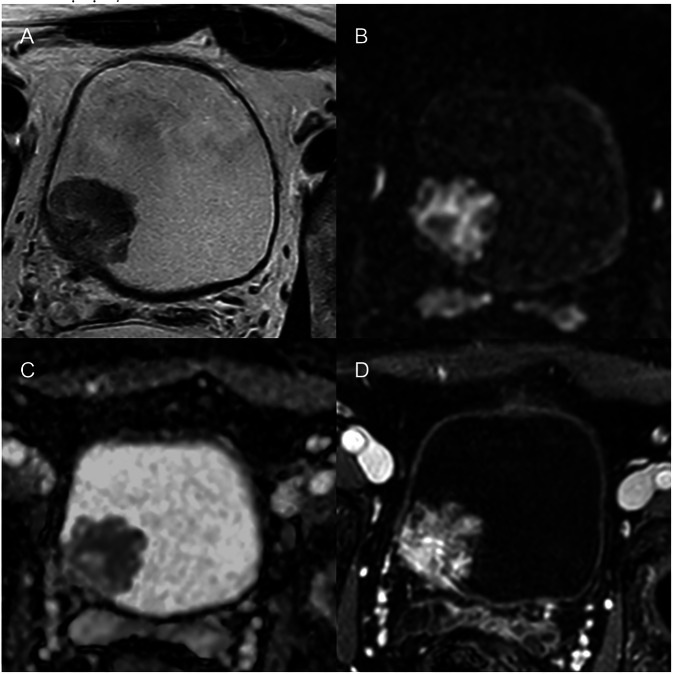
MRI in a 57-year-old male with bladder cancer. T2-weighted (A), DWI (B), ADC map (C), and post-contrast (D) images show a 3 cm lesion in the right posterior wall of the bladder with infiltration of the muscularis propria, consistent with VI-RADS 4
